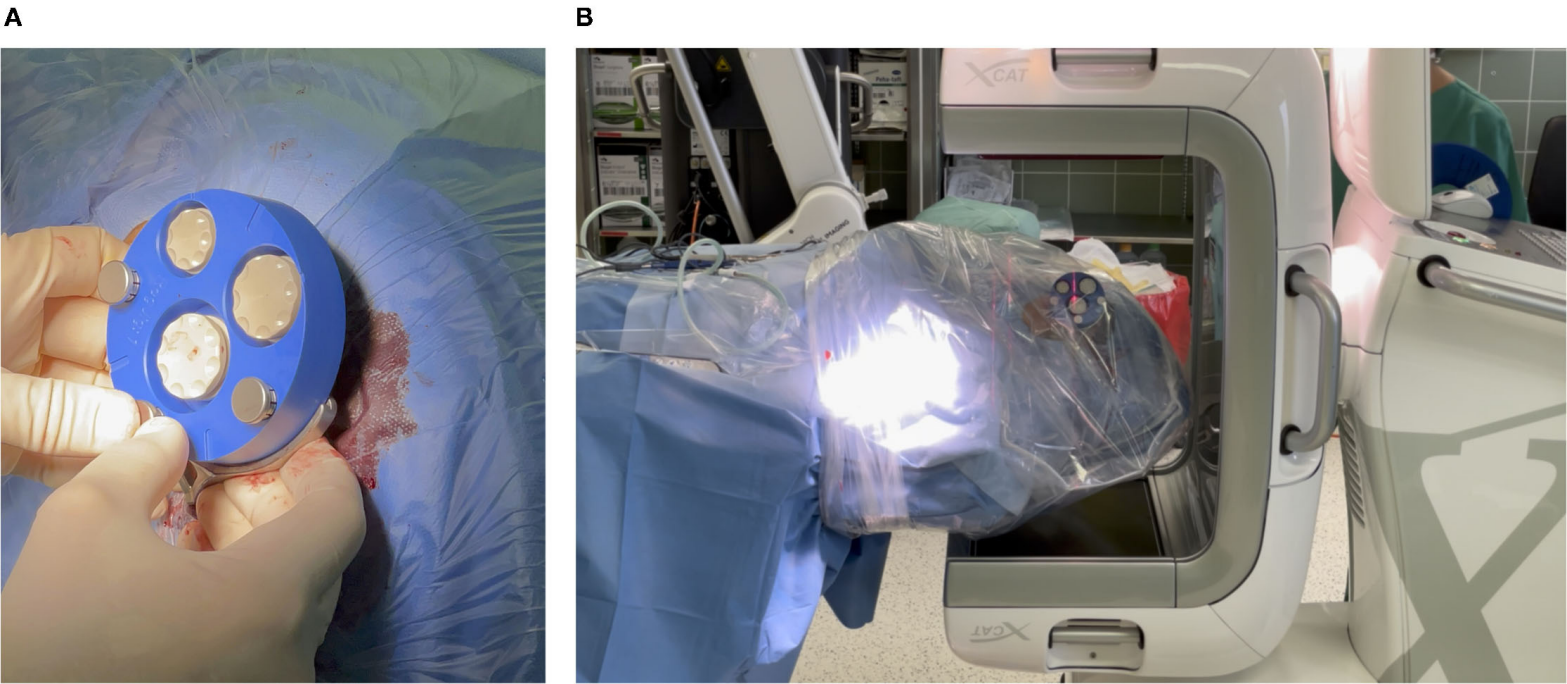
(A) Mounting X-ray marker (blue). The X-ray marker has dense markers embedded, which help to localize the markers in the imaging and thereby the position of the frame can be determined. (B) Acquiring a cone beam computed tomography (CBCT) scan. To avoid contamination, the patient's head is covered with sterile foil.
